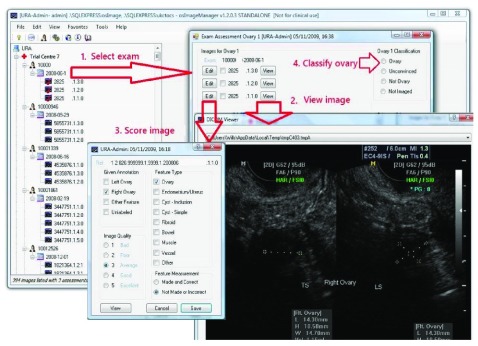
Screenshots of the osImageManager – the application used to facilitate Transvaginal Ultrasound Exam Review.
